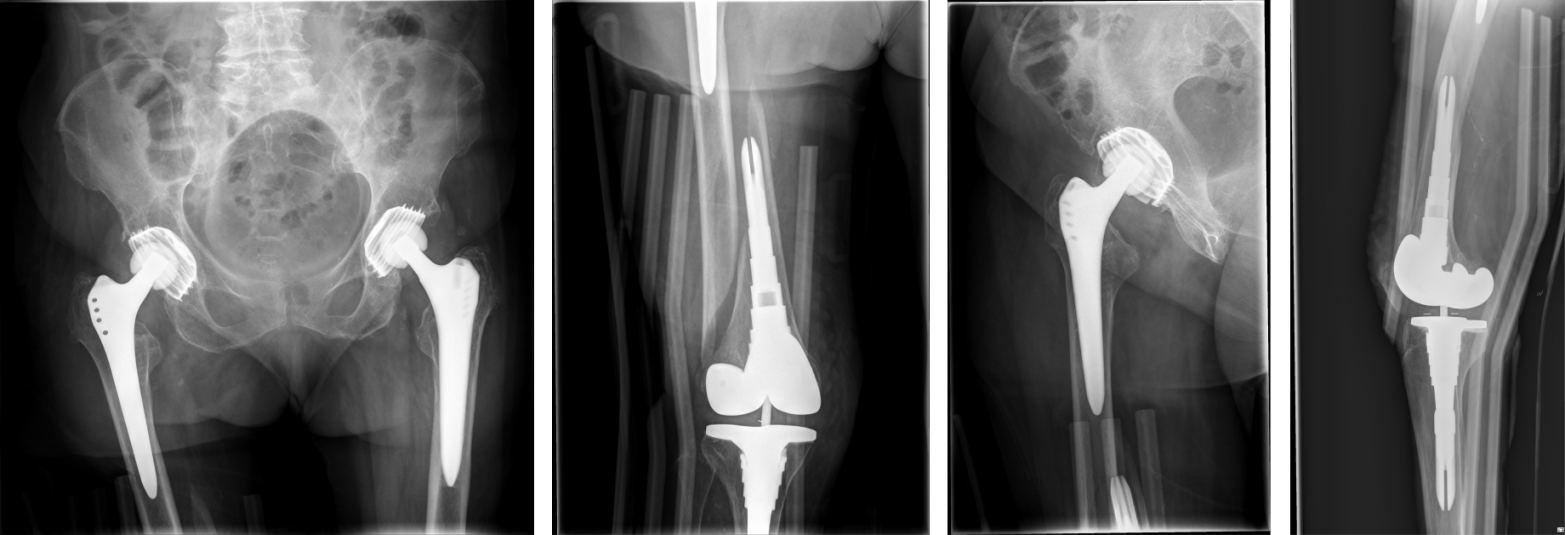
X-ray of the right thigh with the adjacent joints performed prior to surgery using the custom made endoprosthesis
